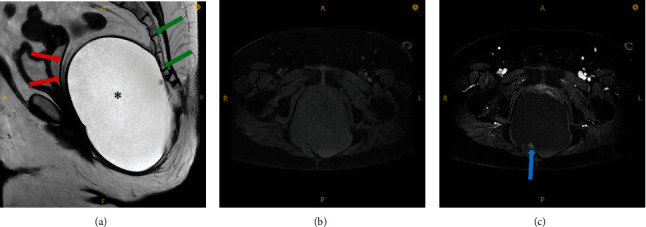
Pelvic MRI of the tumor showing a large retrorectal cystic mass with solid mural nodule. A sagittal T2 weighted pelvic MRI (a) demonstrated a large predominantly cystic mass (∗) which is anterior to the sacrum and coccyx (green arrow) and posterior to the rectum (red arrow). An unenhanced fat suppressed T1 weighted axial image (b) and a gadolinium enhanced fat suppressed T1 weighted axial image (c) demonstrated an enhancing nodule (blue arrow) in the posterior portion of the mass.
